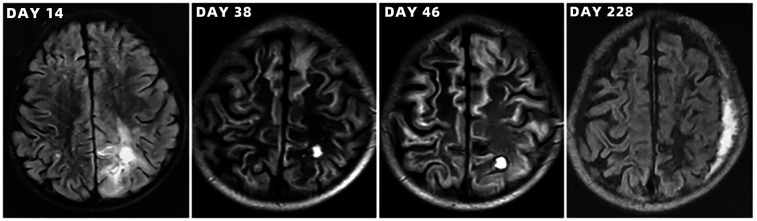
Brain magnetic resonance imaging scans of the toddler during hospitalization and follow-up.
